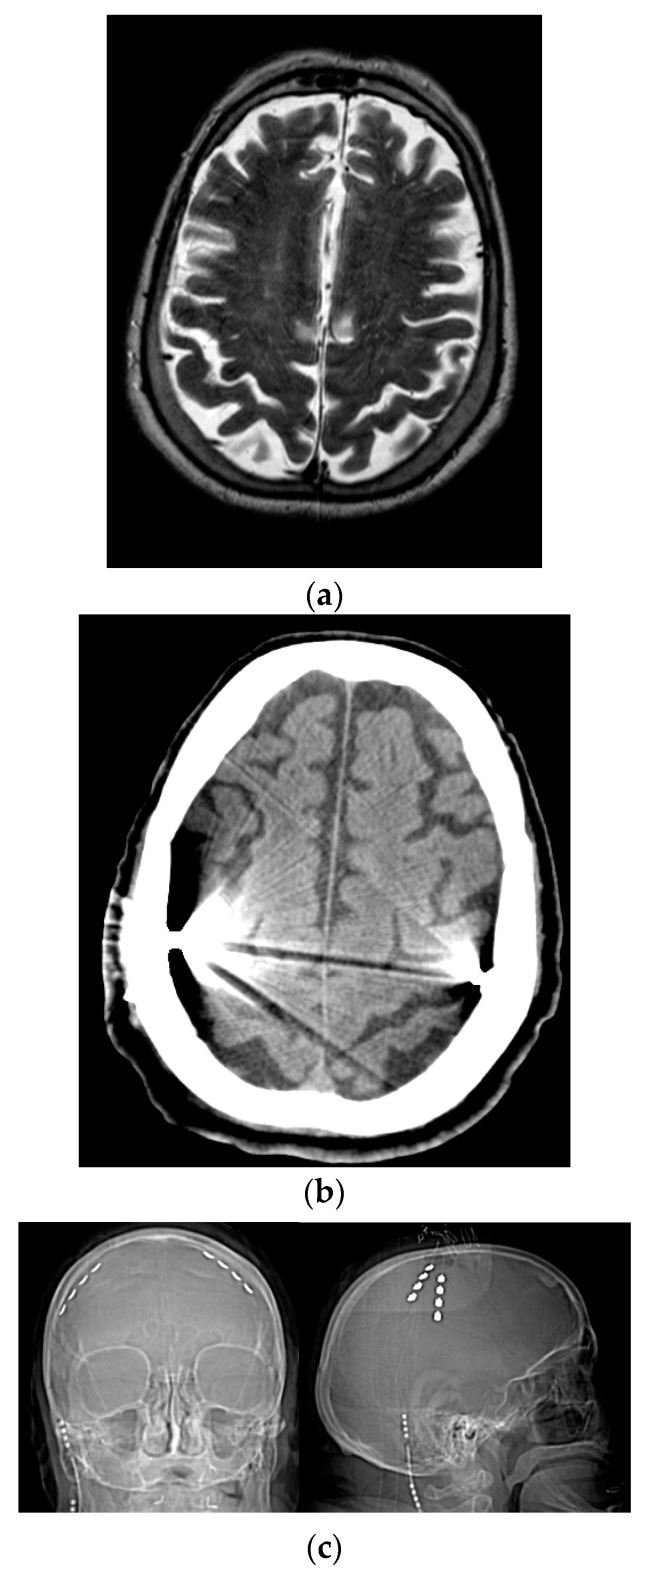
Placement of quadripolar electrode strips over the motor cortices. (a) Preoperative brain MRI. (b) Postoperative brain CT scan. (c) Postoperative skull X-ray. R = right; L = left.
